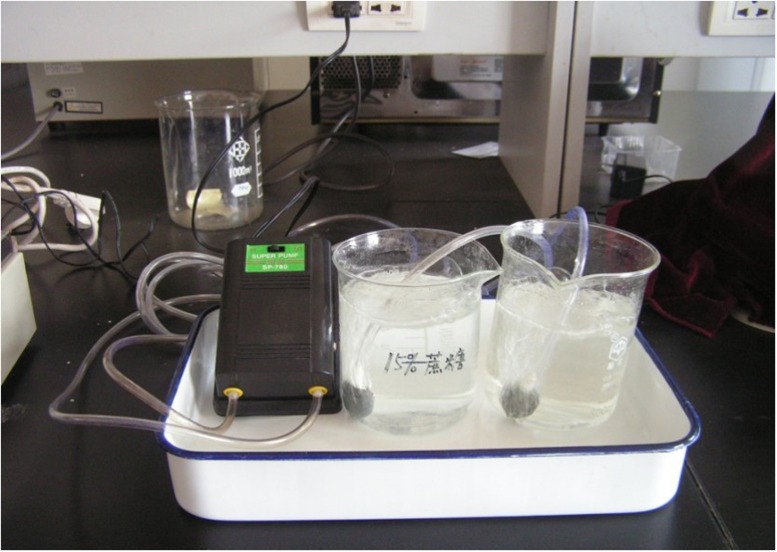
Aeration treatment of sucrose solution for suspending maize pollen. Sieved pollen was suspended in 15% sterilized sucrose solution in a beaker and the suspension was aerated by an air pump for 20 min prior to ultrasonication treatment.
